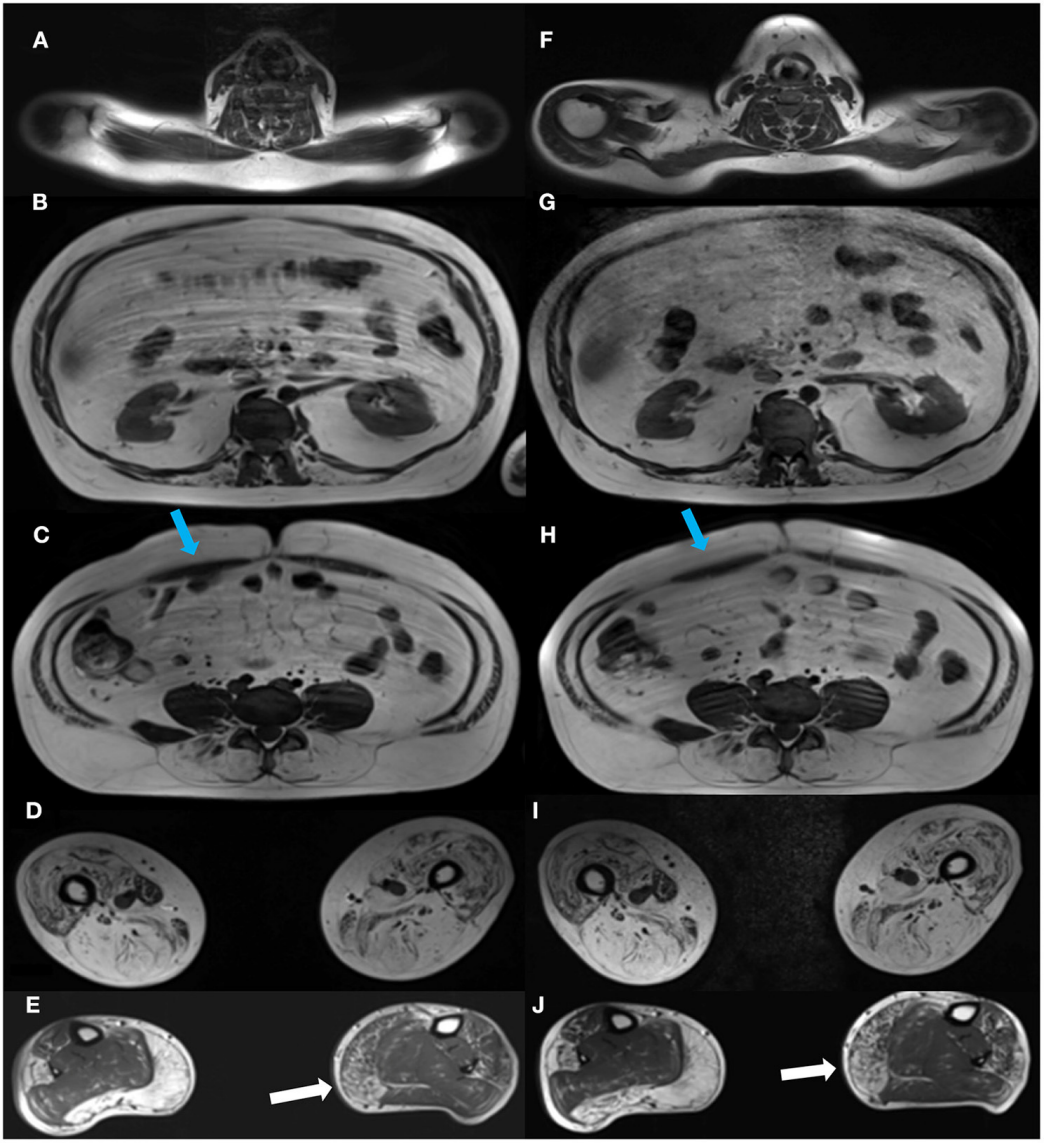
Cross-sectional MR images at baseline and at follow-up. Images of the cross-sectional slices at baseline (left column, A–E) and at follow-up (right column, F–J). Images are shown at spinal levels C6 (A,F), T12 (B,G), and L4/L5 (C,H), thighs (D,I), and lower legs (E,J). A visual inspection of the images shows some progression of the right medial head of gastrocnemius from baseline, (E) to follow-up (J), while changes in other muscles from baseline are indiscernible. Blue arrows show the abdominal muscles (C,H).
